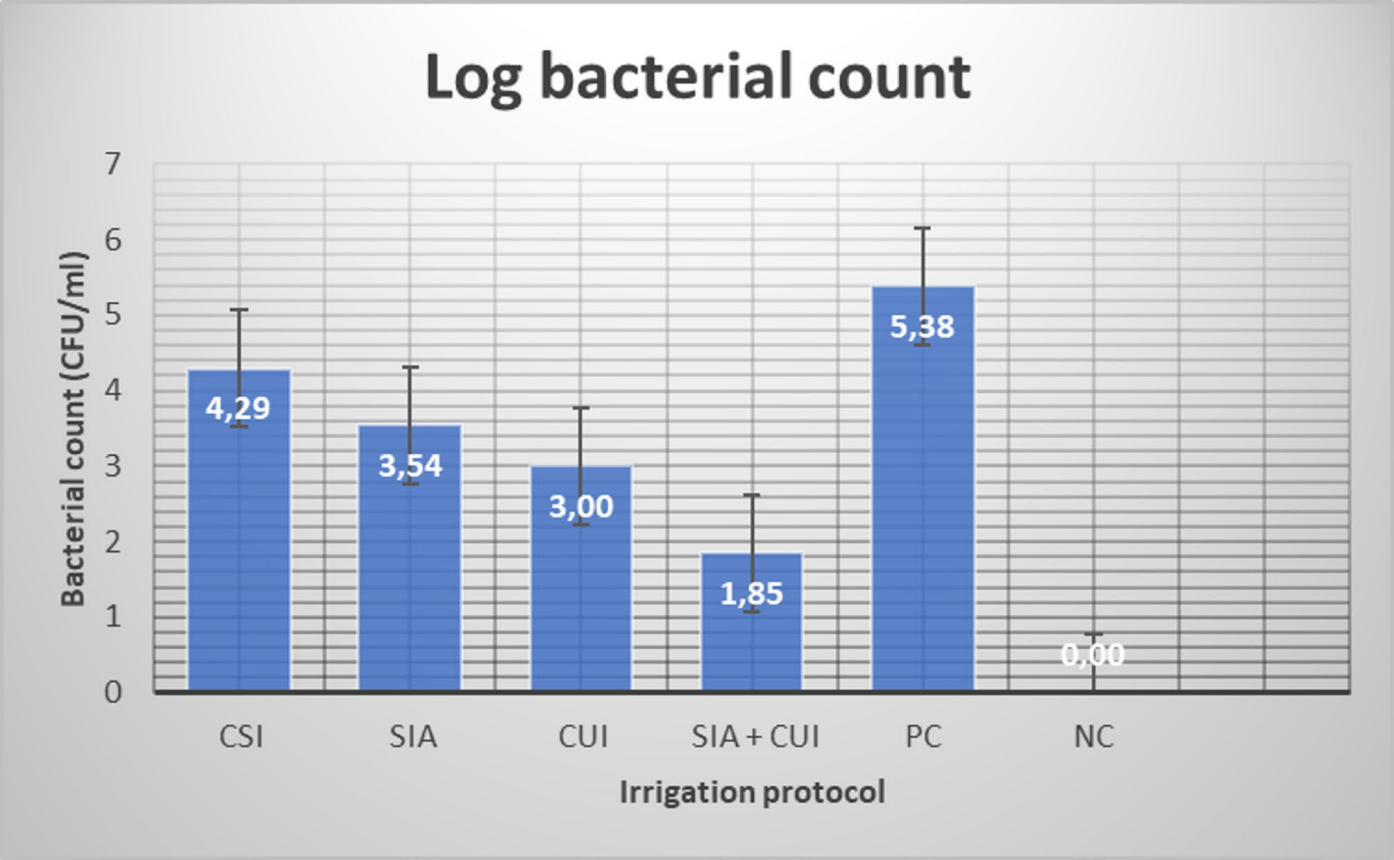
Bacterial count results according to the experimental groups ([CSI] conventional syringe irrigation; [SIA] stepwise intraoperative activation; [CUI] conventional ultrasonic irrigation; [SIA + CUI] stepwise intraoperative activation plus conventional ultrasonic irrigation, [PC] positive control; [NC] negative control groups)
